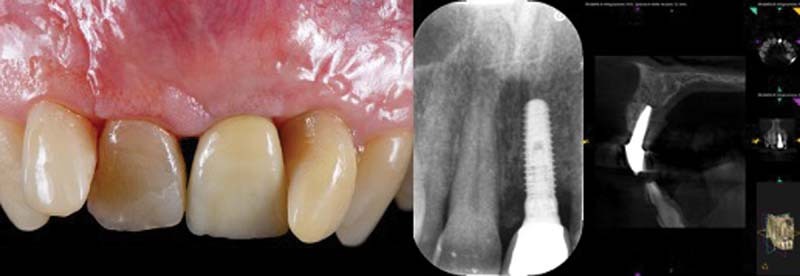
Clinical and radiographic (2D, 3D) follow up at 1 year. 2D, two-dimensional; 3D, three-dimensional.
